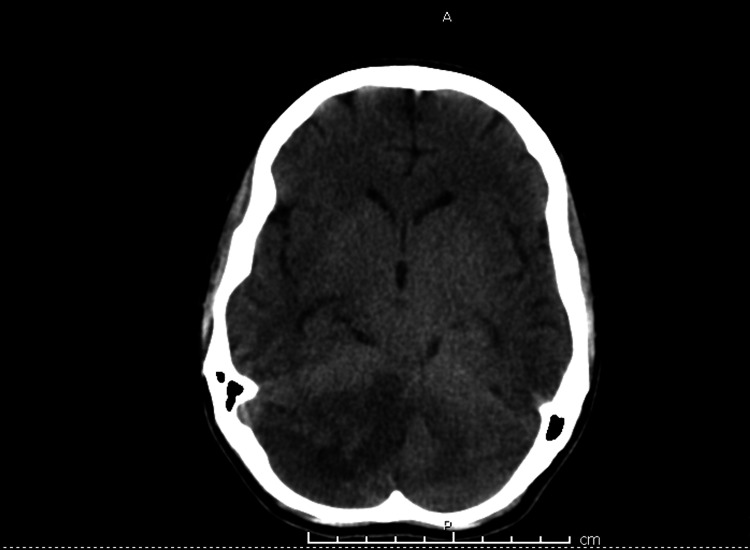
Brain CT showing cerebellar hemorrhage due to septic emboli
